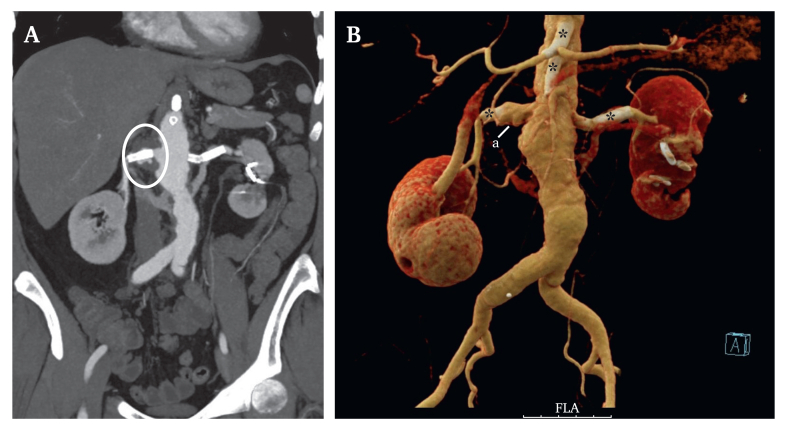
(A) Pre-operative computed tomography angiography of the aorta showing a penetrating aneurysm at the origin of the right renal artery with the dislocated stent. (B) Computed 3D reconstruction of the penetrating aneurysm. The proximal renal artery and the adjacent aortic wall are irregular shaped. (a) Renal artery; stars, occluded stents in both renal arteries, the coeliac trunk, and the superior mesenteric artery.
